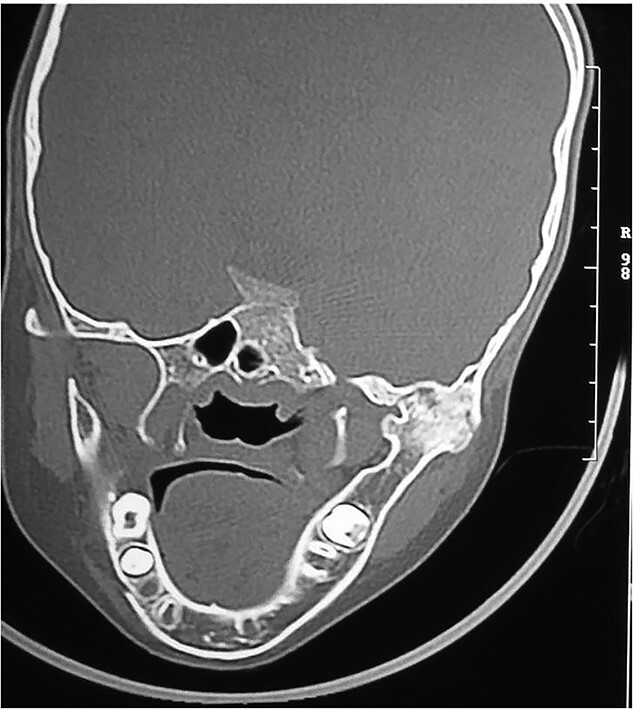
Right side bony ankylosis in coronal section CT.
